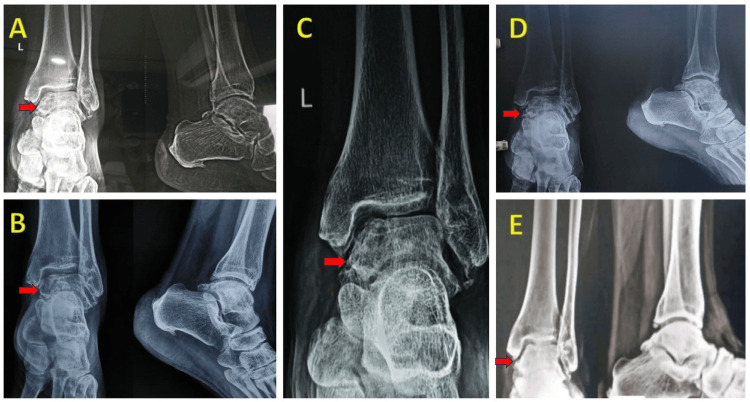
Sequential Radiographs Serial yearly follow-up x-rays showing good integration of bone graft with some signs of degenerative changes and no recurrence. Figure 4A - 2017, Figure 4B - 2018, Figure 4C - 2019, Figure 4D - 2021, Figure 4E - 2022.
